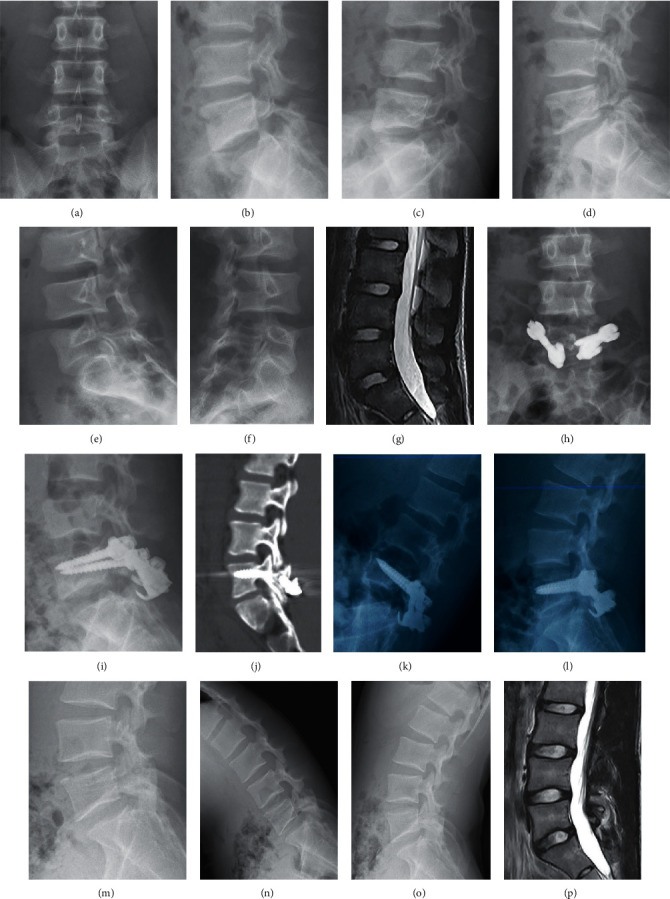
A 16-year-old male patient with LGIS of L5 had LBP of 7 points (VAS scores), without sciatica pain, and underwent direct pars repair using the PSRH system. (a)–(f) The X-rays of the lumbar spine in anteroposterior, sagittal, hyperextension and flexion, and double oblique positions, suggesting LGIS of the L5 with isthmic defect. The disc height and ROM of L5-S1 are 12.95 mm and 23.9°, respectively. (g) The surgical segment and the upper segment are both grade 2 of Pfirrmann's criteria by magnetic resonance imaging (T2-weighted MRI). (h)-(i) The postoperative X-rays, indicating that the isthmic defect has been repaired and lumbar 5 spondylolisthesis has been reset. (j) CT scan of the lumbar spine 12 months after surgery, showing that the isthmic defect has bony fusion. (k)-(l) X-ray films of hyperextension and flexion before removal of internal fixation at 42 months after the operation. (m)–(o) Re-examinations of the lumbar spine X-ray at 78 months after the operation, suggesting that the internal fixation has been removed. The disc height and ROM of the affected level were 10.6 mm and 16.6°, respectively, and the ROM of the upper segment was 12.10°. (p) The lumbar spine MR at the FFU was grade 1 for the L4-5 discs and grades 2-3 for L5-S1 discs.
